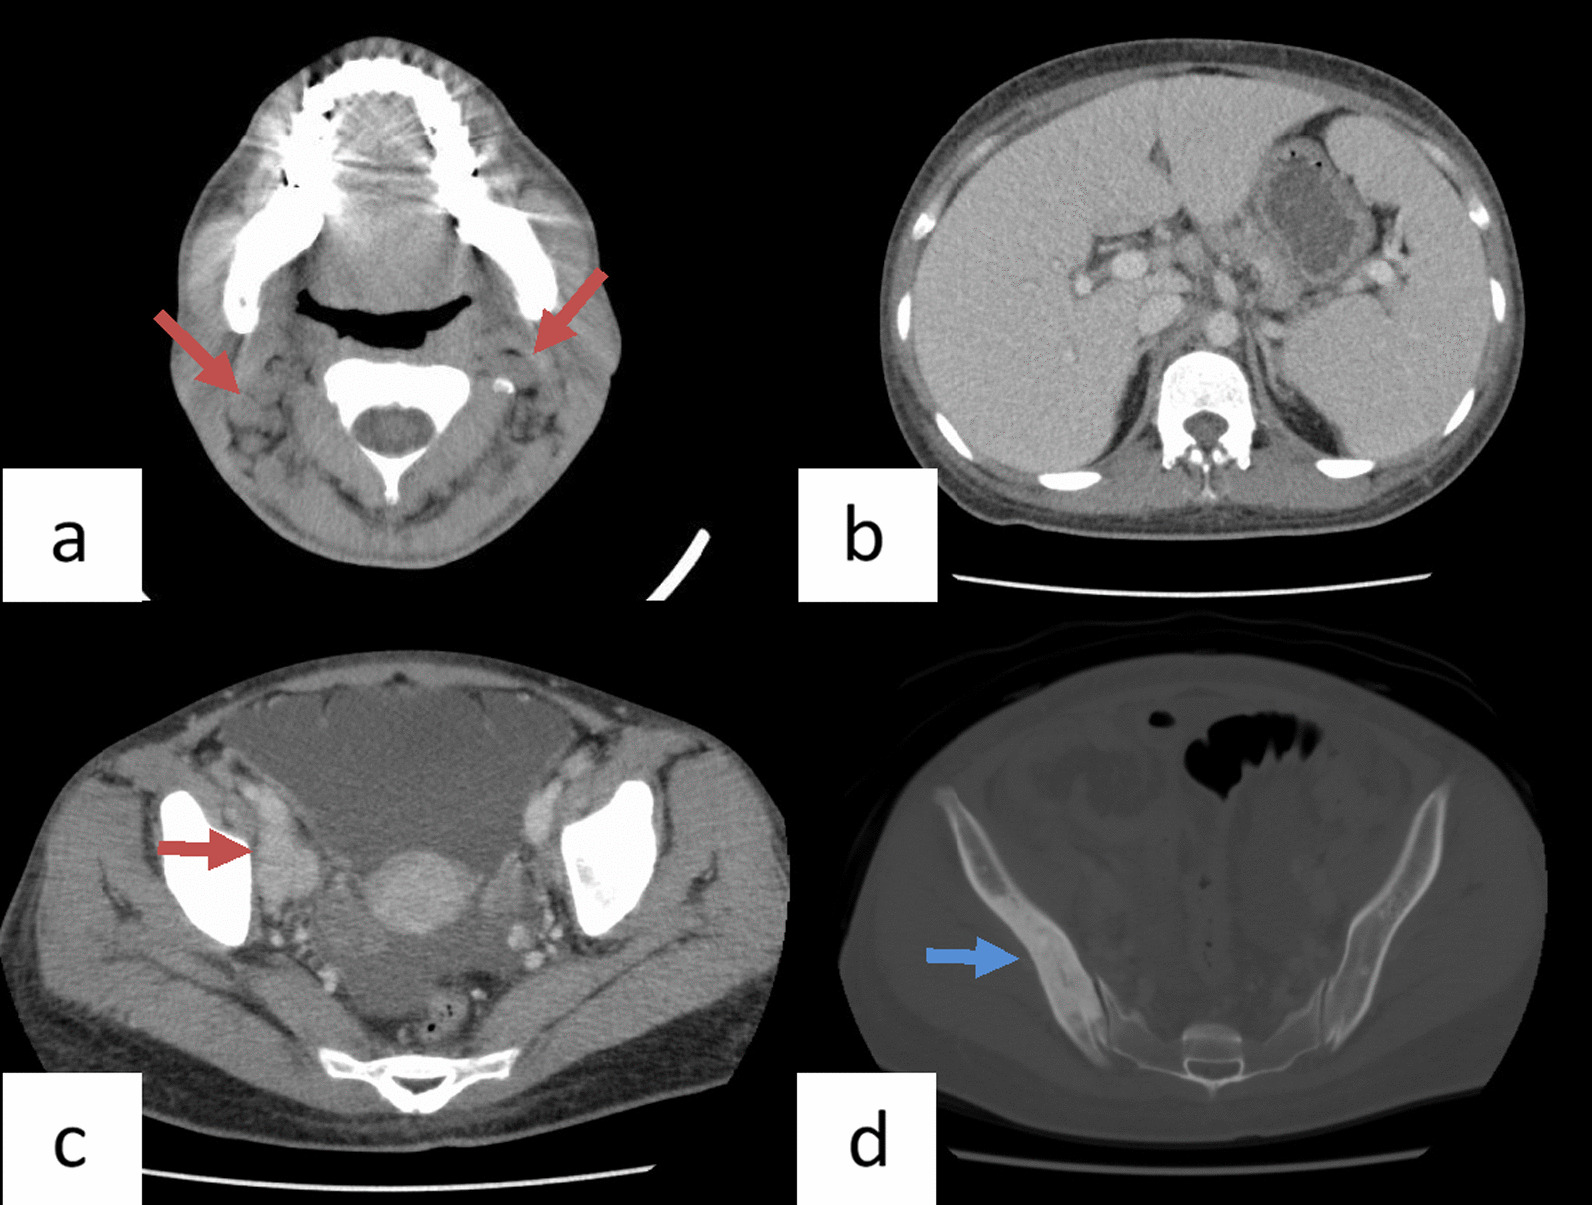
Computed tomography before treatment. a Cervical lymphadenopathy (reddish brown arrows); b hepatosplenomegaly; c right external iliac lymphadenopathy (reddish brown arrow) and ascites; d osteosclerosis of the ilium (blue arrow)
